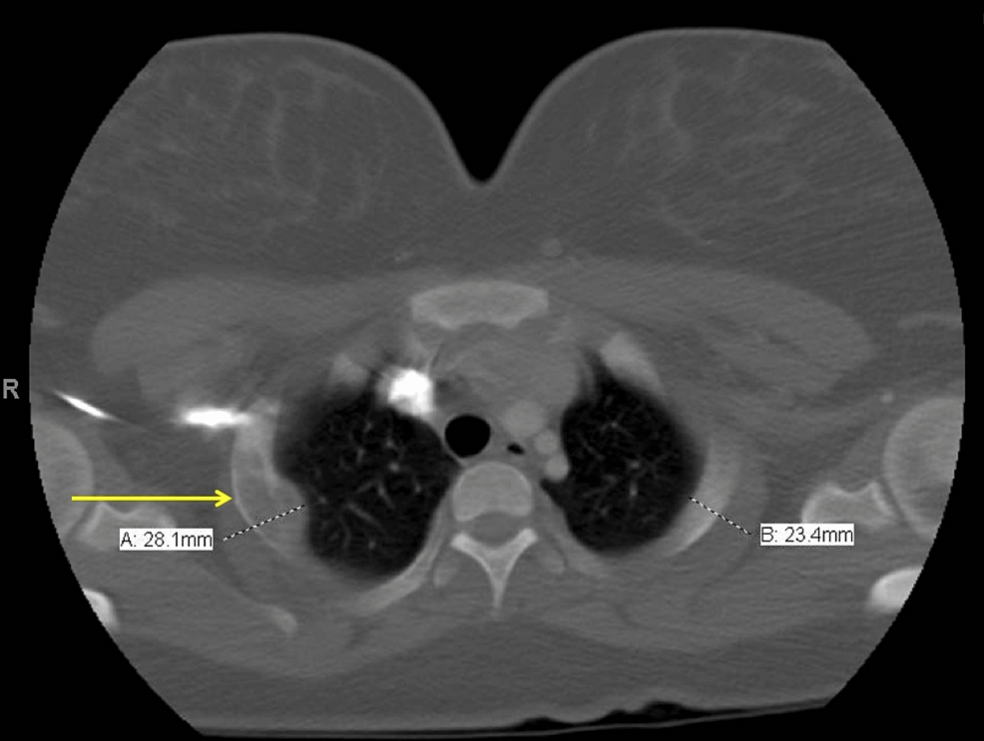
Chest CT with a focal lytic lesion of the posterolateral right second rib (arrow) with small extra-pleural-based soft tissue density. CT, computed tomography
